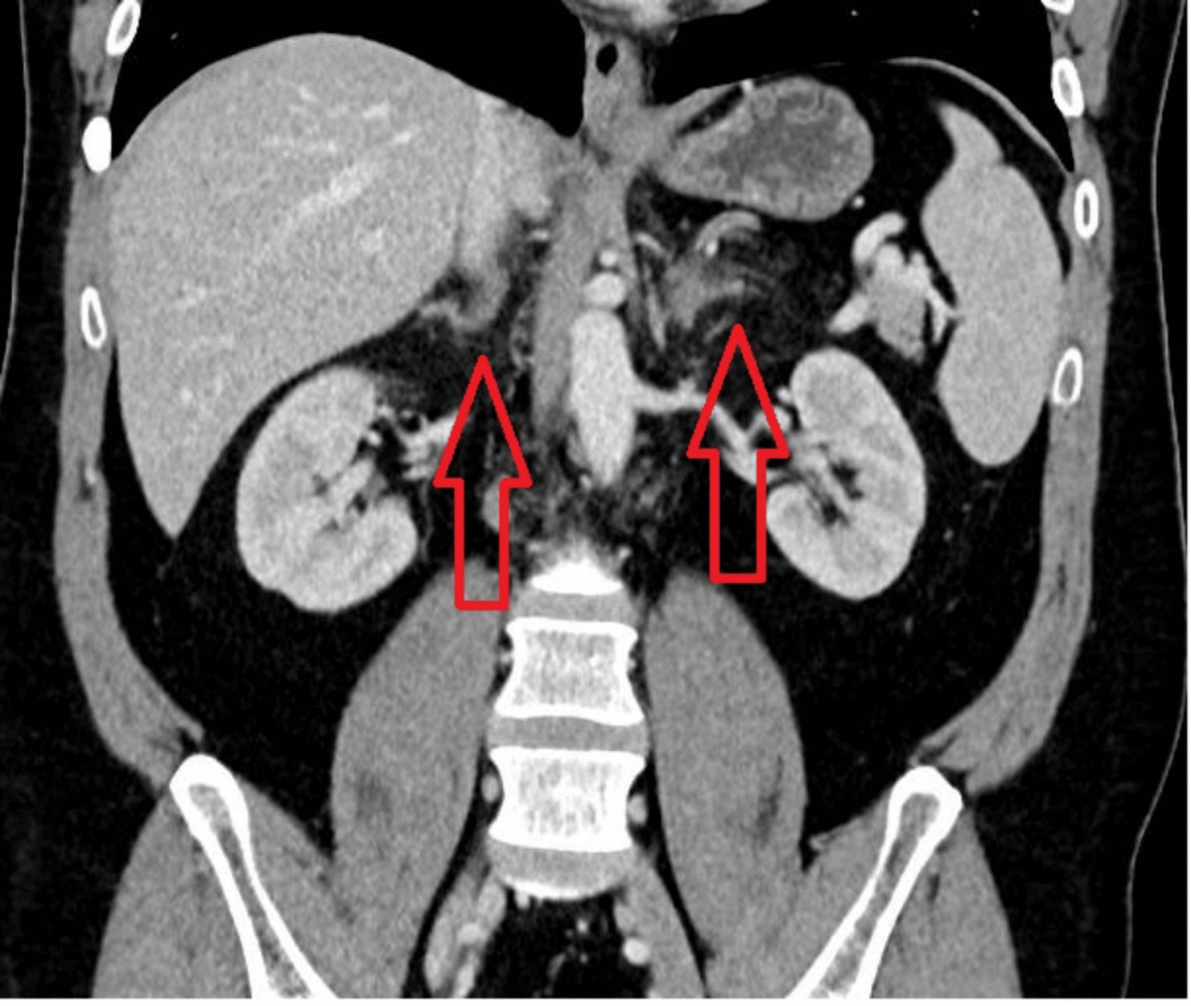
Coronal contrast-enhanced CT image demonstrating bilateral adrenal enlargement (arrows) and periadrenal fat stranding without evidence of hemorrhage
